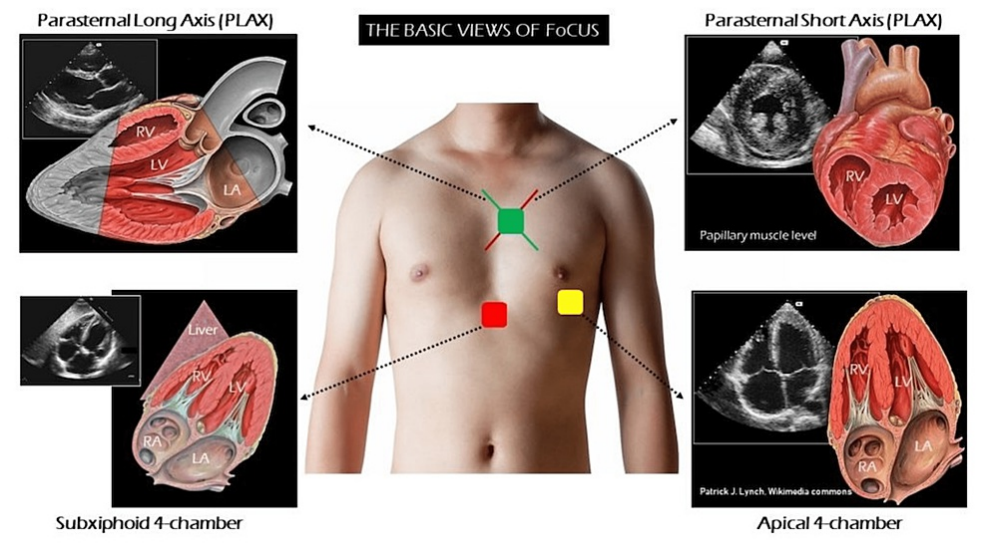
The common views of POCUS trans-thoracic echocardiography Source: Renal Fellow Network [14]. Reprinted with permission. POCUS: Point-of-Care Ultrasound
